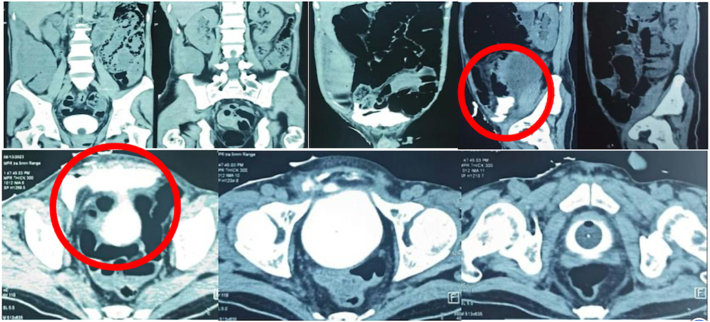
CT Cystography Examination.
